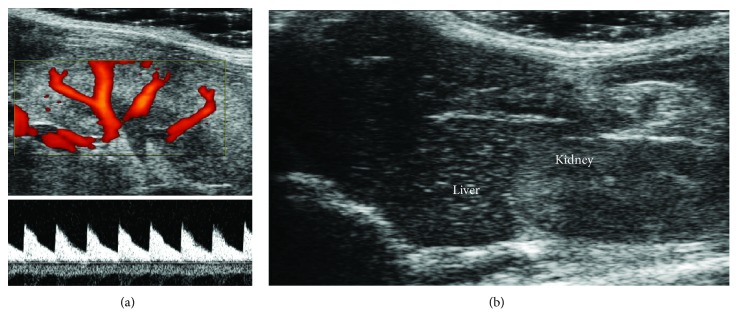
Kidney and liver ultrasound scan. (a) Power Doppler image was used to localize renal vessels, while PW-Doppler signal was used to measure renal blood flow thus deriving pulsatility and reflectivity indexes. (b) Mean gray levels of the liver parenchyma were compared to mean gray levels of the renal parenchyma.
